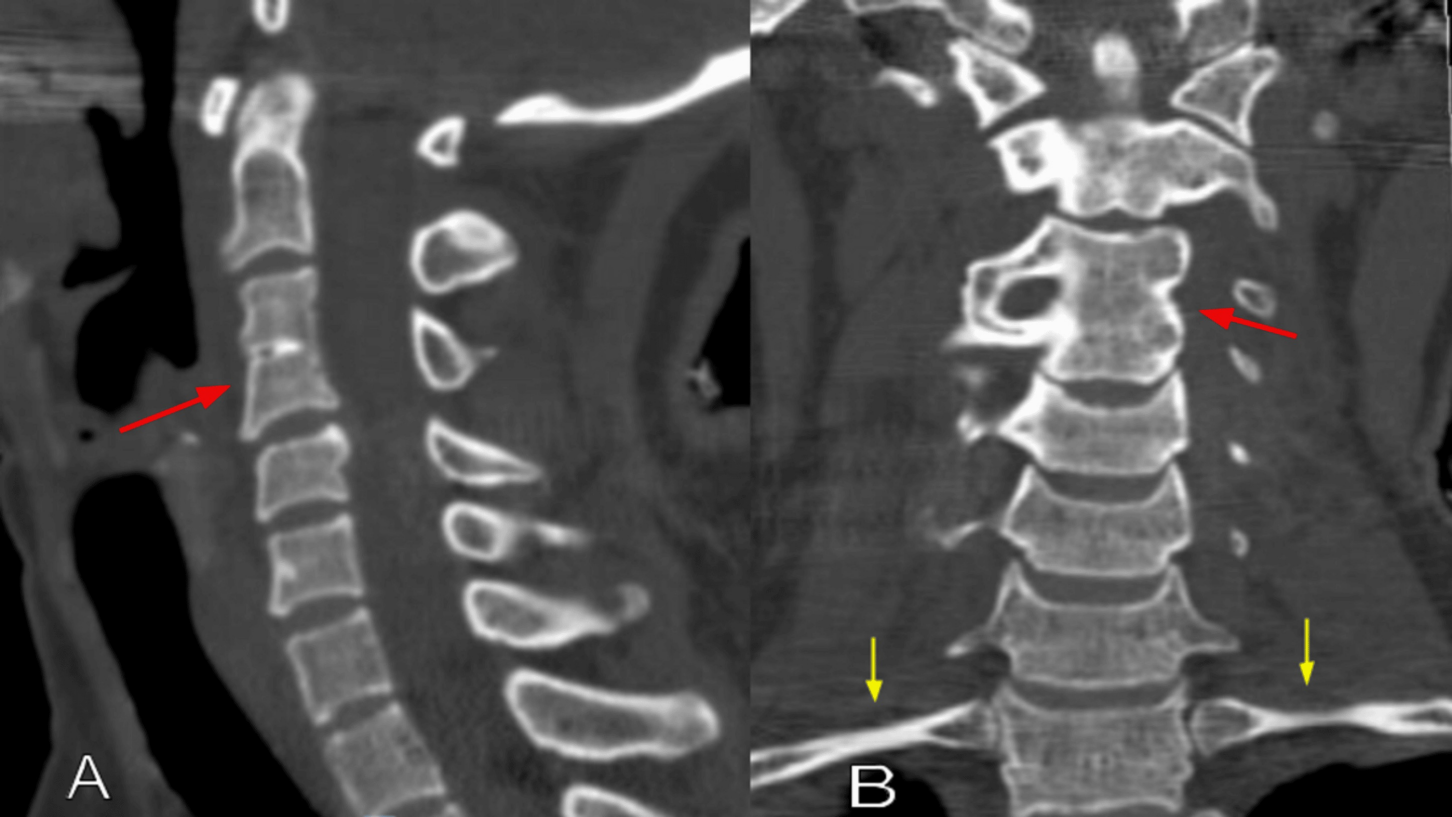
Cervical CT scan bone window (A) sagittal and (B) coronal images showing a C3-C4 vertebral body fusion (red arrows), with intact first ribs (yellow arrows).
